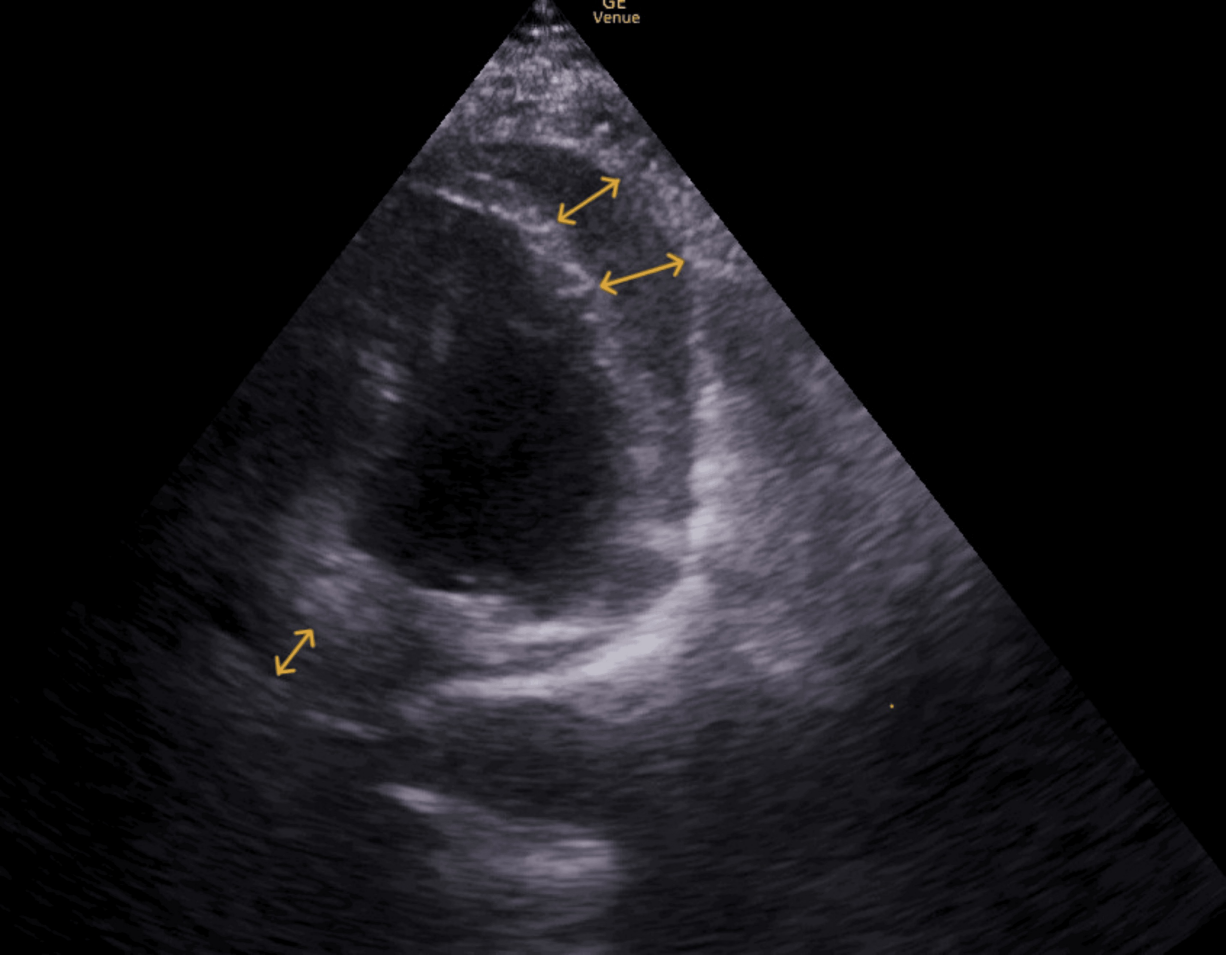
Four-chamber cardiac ultrasound. Cardiac POCUS clearly shows the significant pericardial effusion (arrows). POCUS: point-of-care ultrasound
